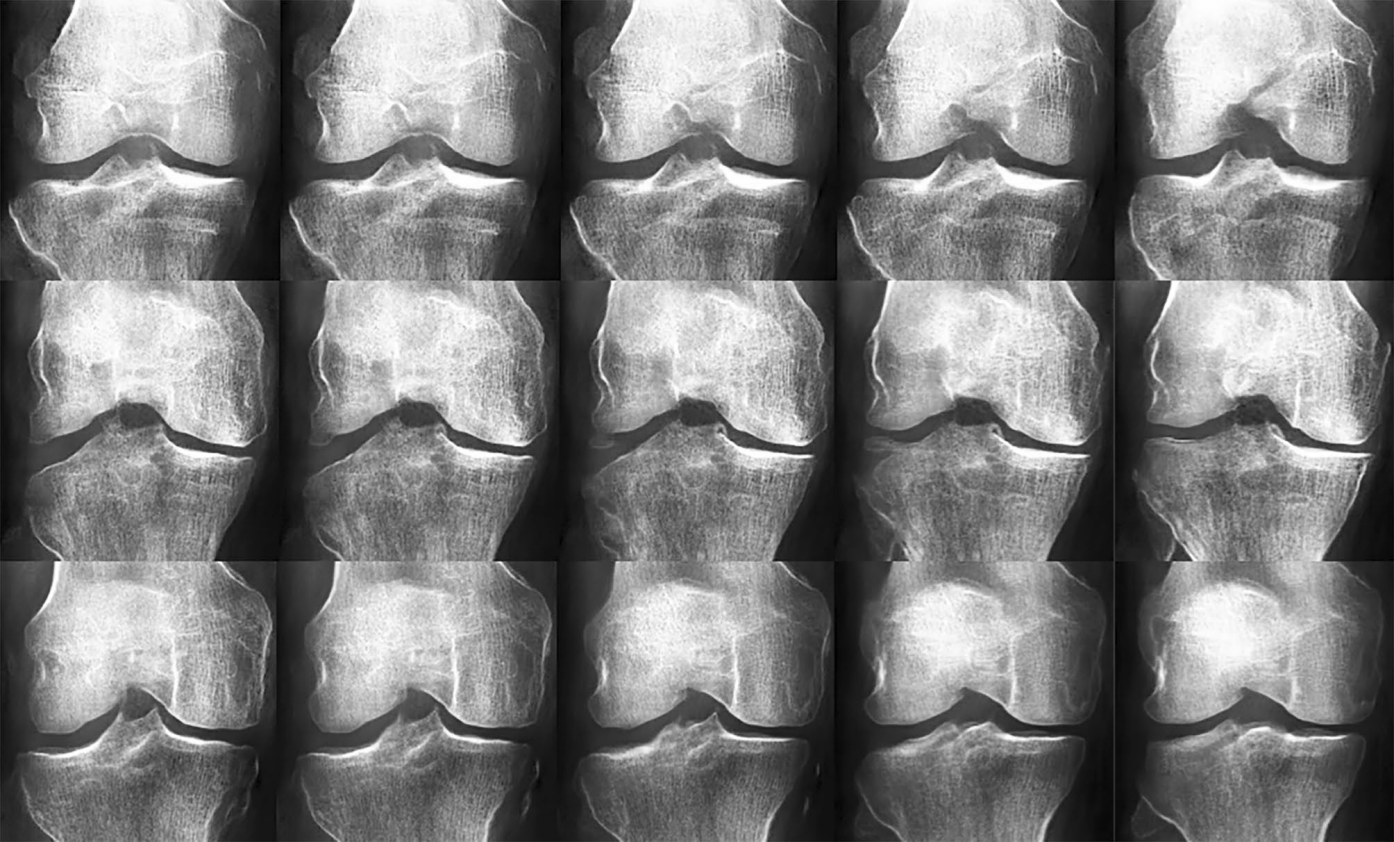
Latent dimension perturbations are displayed. The first two rows show changes in one latent dimension. Left to right for positive change, and vice versa for negative change. The last row showcases linear interpolation between two X-ray latent space coordinates at the extremes of the row.
